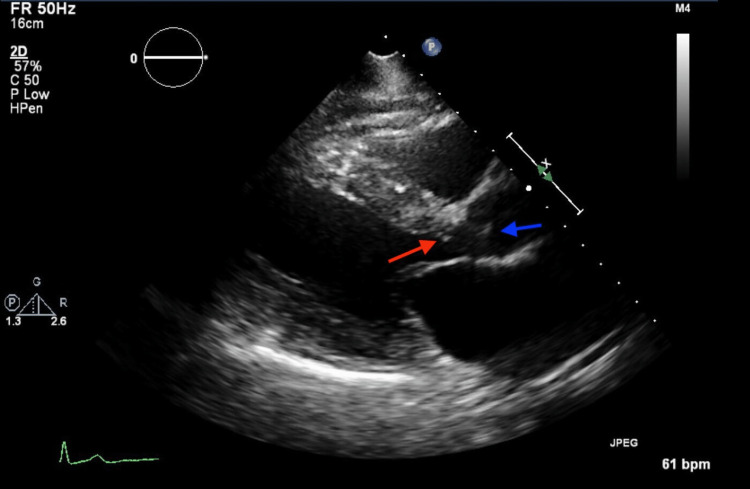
Transthoracic echocardiogram (parasternal long axis view) showing subaortic membrane (red arrow) and aortic valve leaflets (blue arrow).
